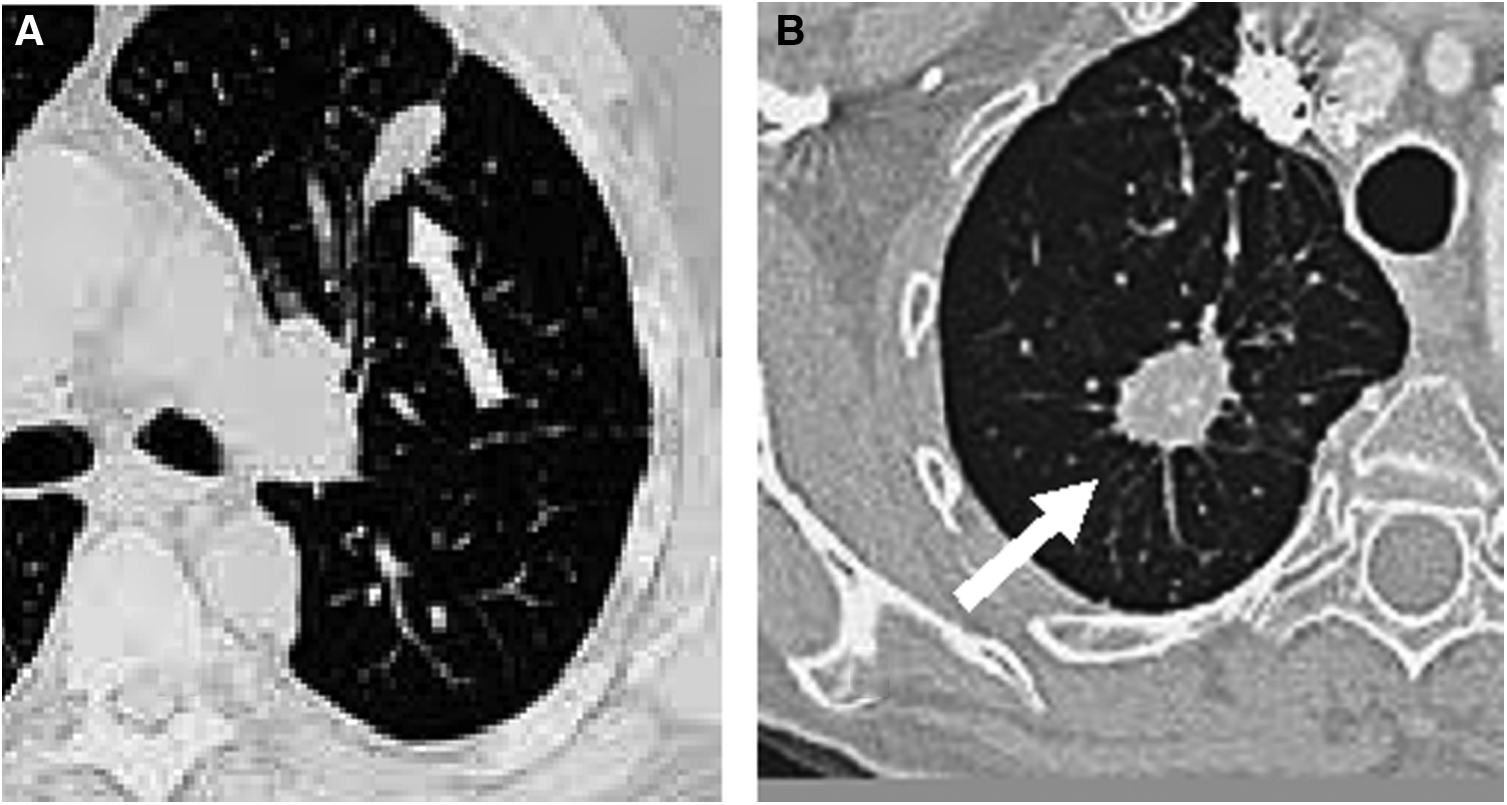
Examples of nodules on CT slices, (A) benign and (B) malignant.
